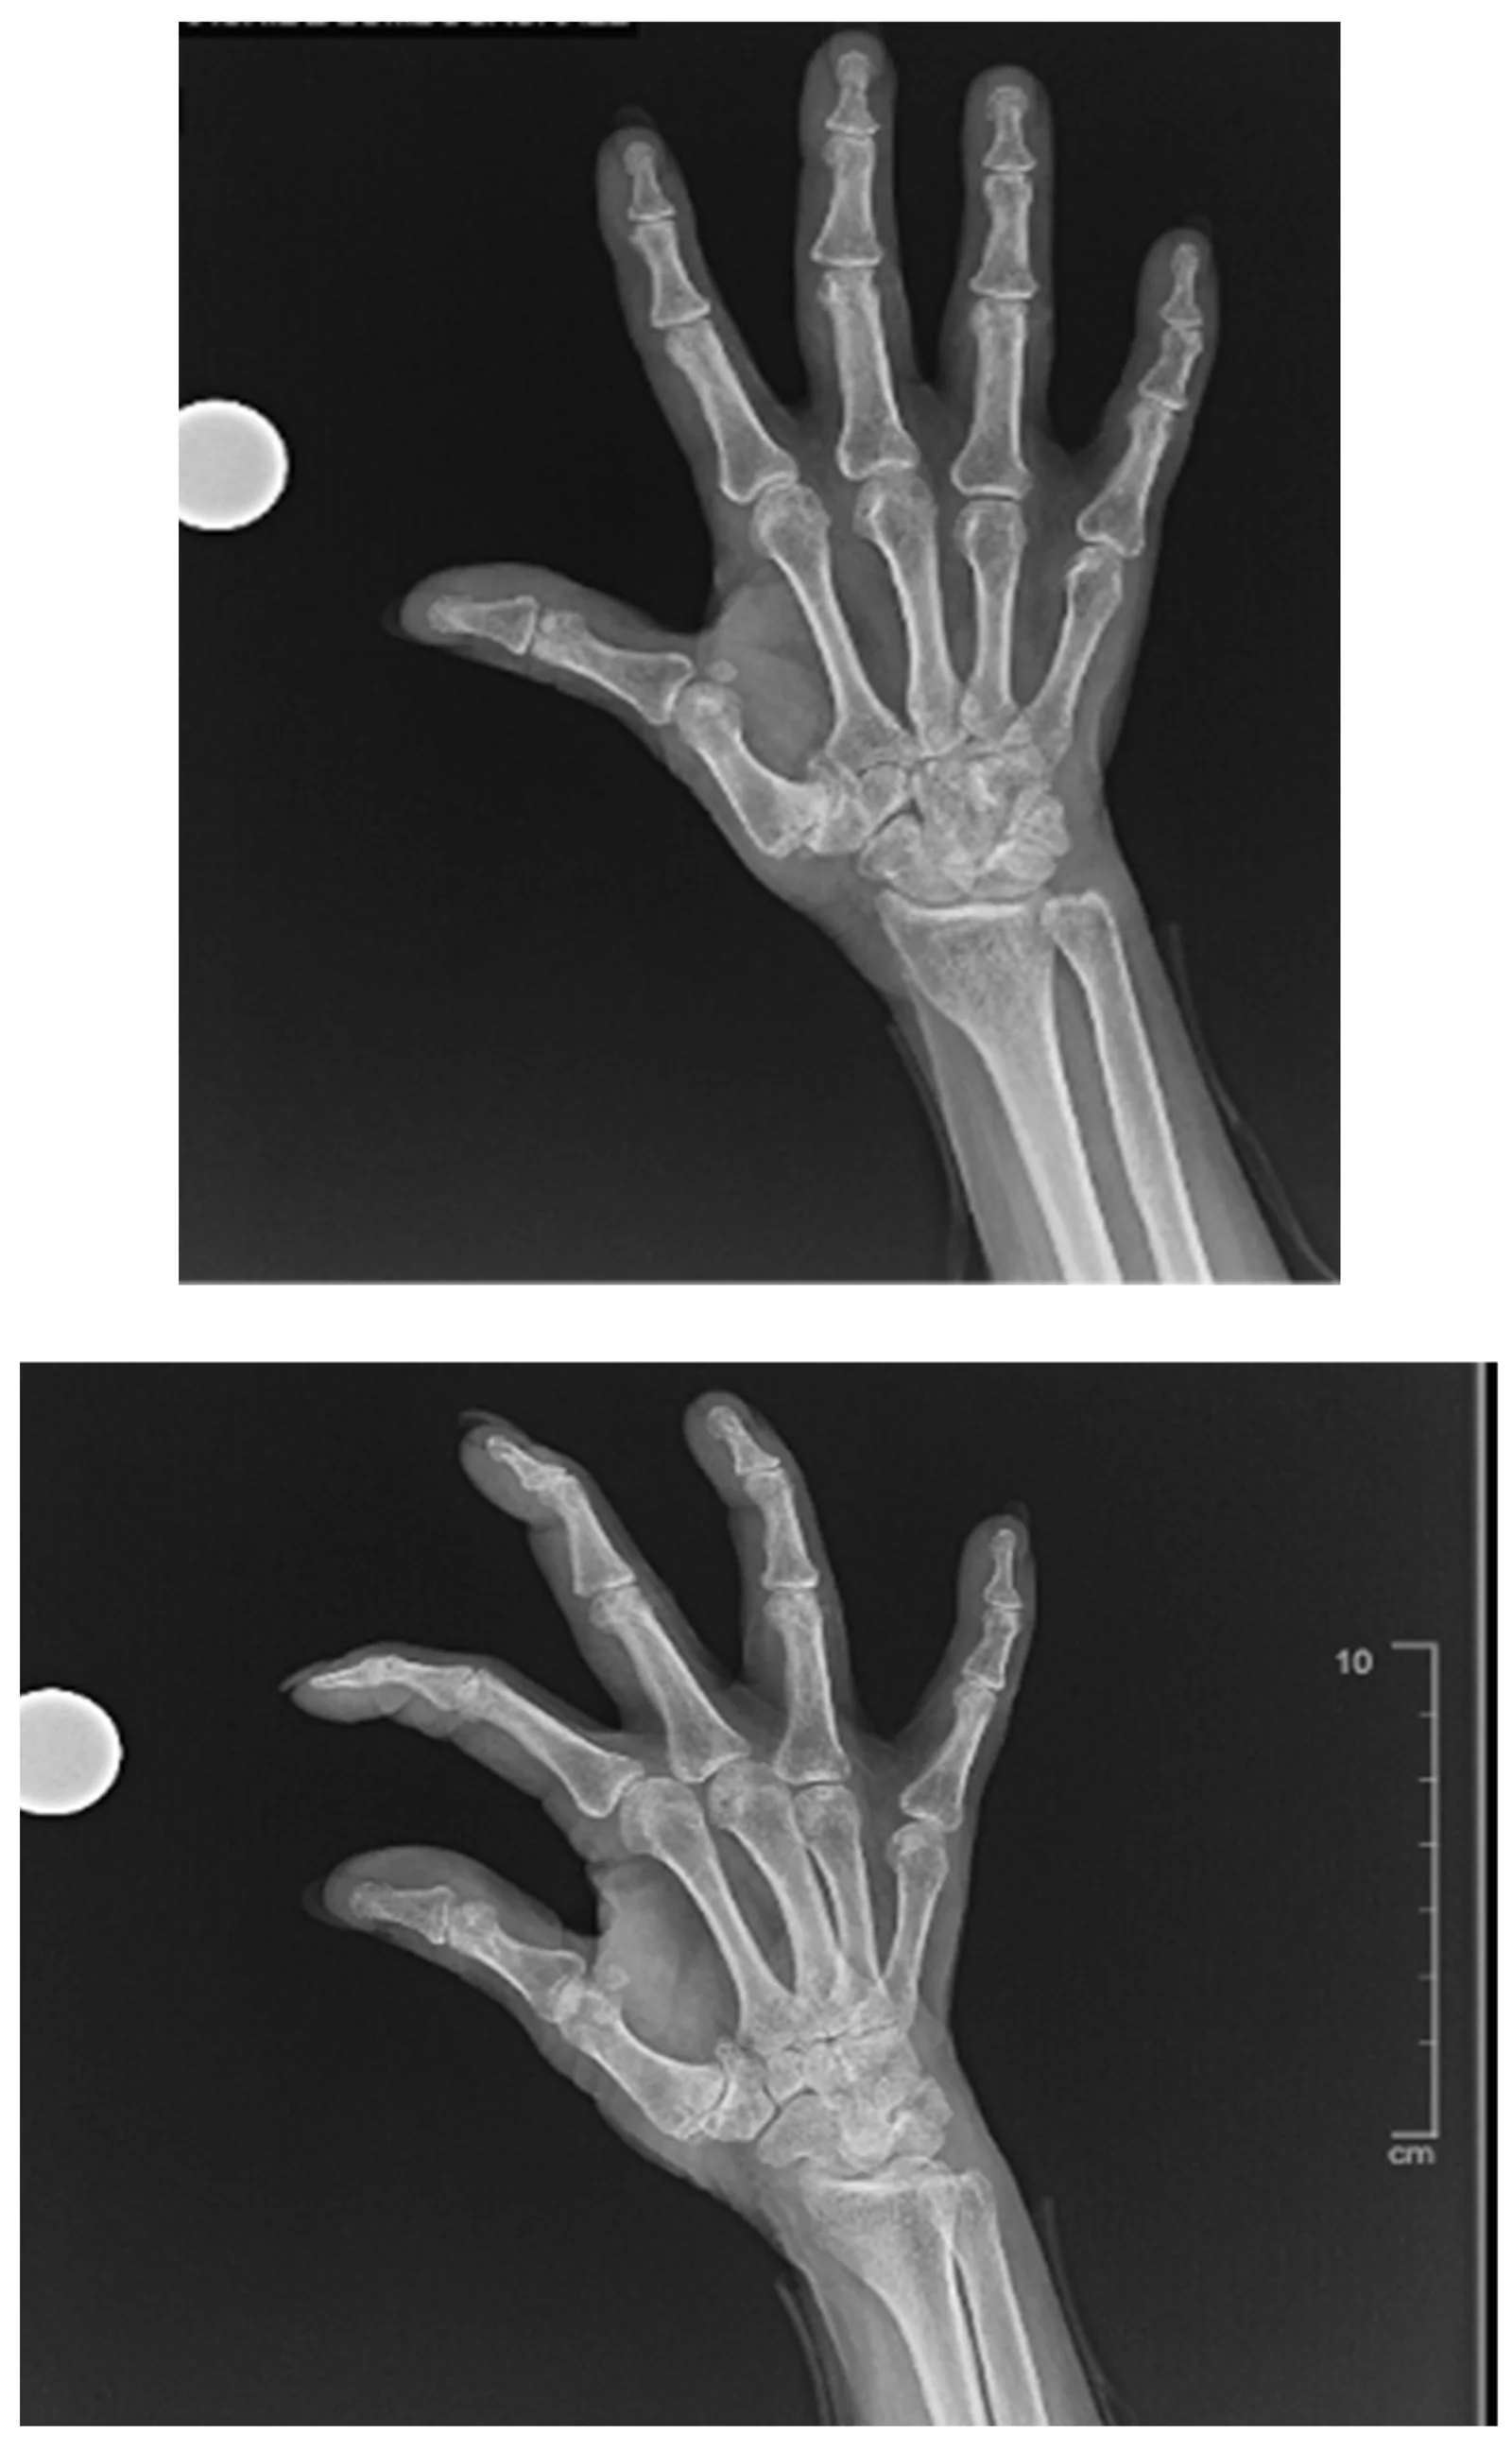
Preoperative radiograph obtained with a 2-Euro coin as a calibration marker.
